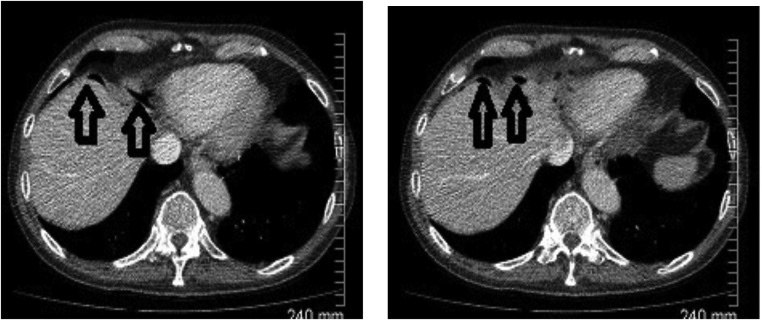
Contrast-enhanced CT abdomen confirming pockets of free intraperitoneal air just anterior to the liver and close to the gastric antrum (vertical arrows).
